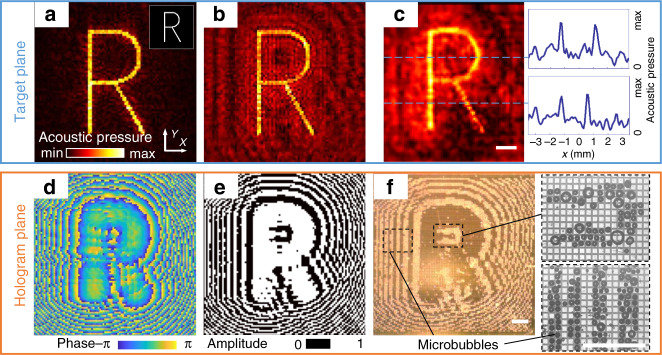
Performance of the 10,000-element spatial ultrasound modulator. Calculation of the phase hologram for the target image (a) and the corresponding phase front in the plane of the hologram (d). Calculations are based on the iterative angular spectrum method, and the phase distribution at the hologram plane is modified upon the back-propagation from the target acoustic amplitude field (top right inset). Comparison of the same target in a binary amplitude hologram (b, e). The experimental result showing the hydrophone scan (c) of the acoustic field in the target plane, which is generated by the bubble array in the hologram plane of the SUM (f). All scale bars are 1 mm.
